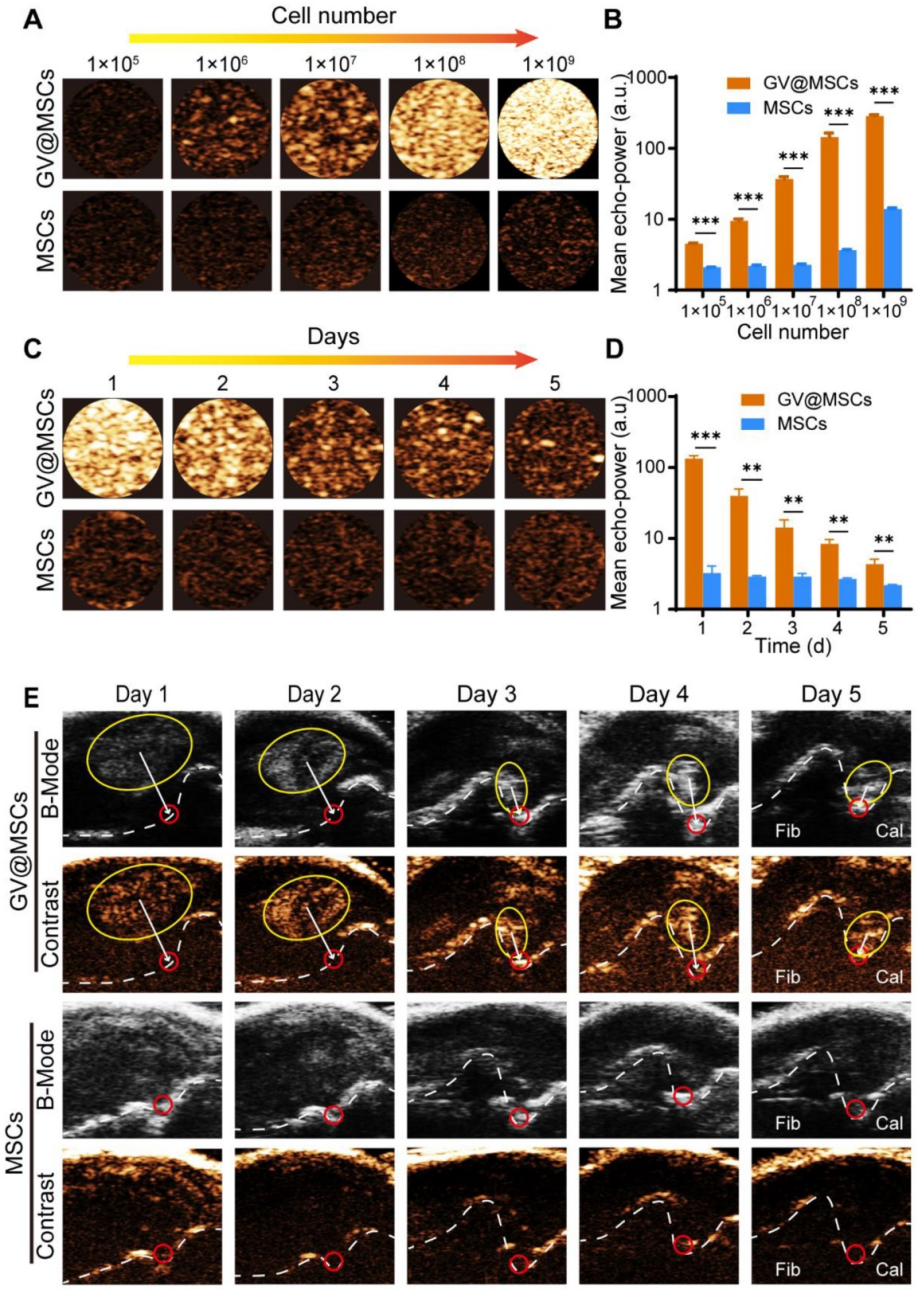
Ultrasound imaging of the GV@MSCs *in vitro* and real-time imaging tracking of the GV@MSCs *in vivo*. (A)
